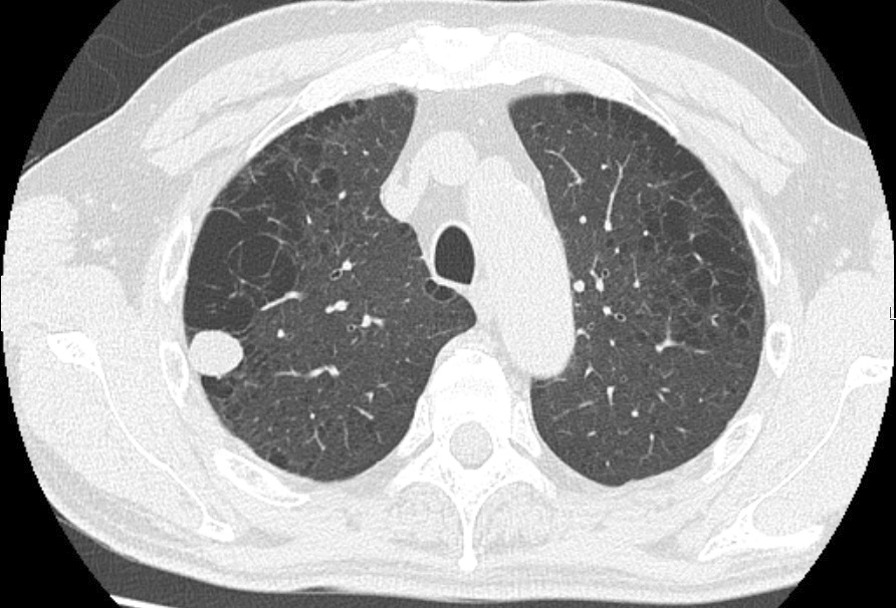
Chest computed tomographic scan showing a 2.0-cm soft-tissue nodule in the right upper lobe of the lung
